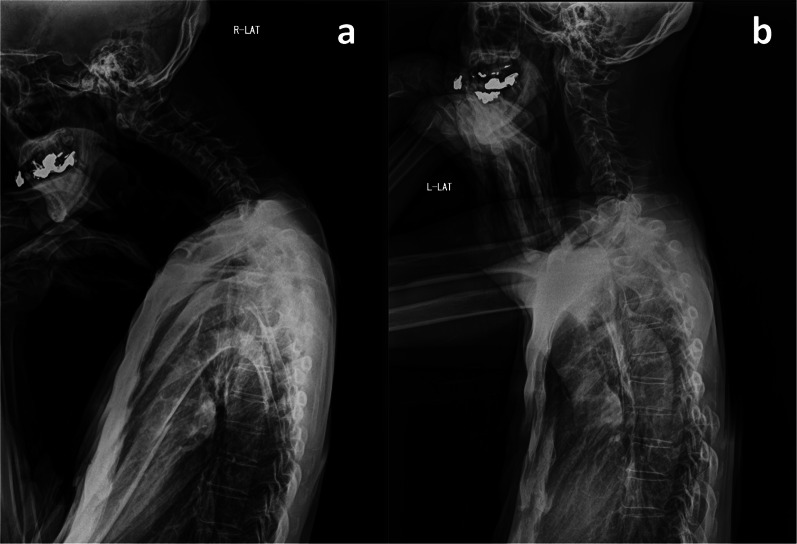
X-ray examination. Cervical–sagittal vertical axis (C-SVA) is defined as the distance between a perpendicular line through the center of the second cervical vertebra and the posterior superior margin of the seventh cervical vertebra. a (POD170): C-SVA was 68 mm; a C-SVA larger than 40 mm indicates an irregular cervical spine alignment. b (POD184): after S-1 + oxaliplatin (SOX) therapy was discontinued, C-SVA improved to 29 mm and his symptoms improved
